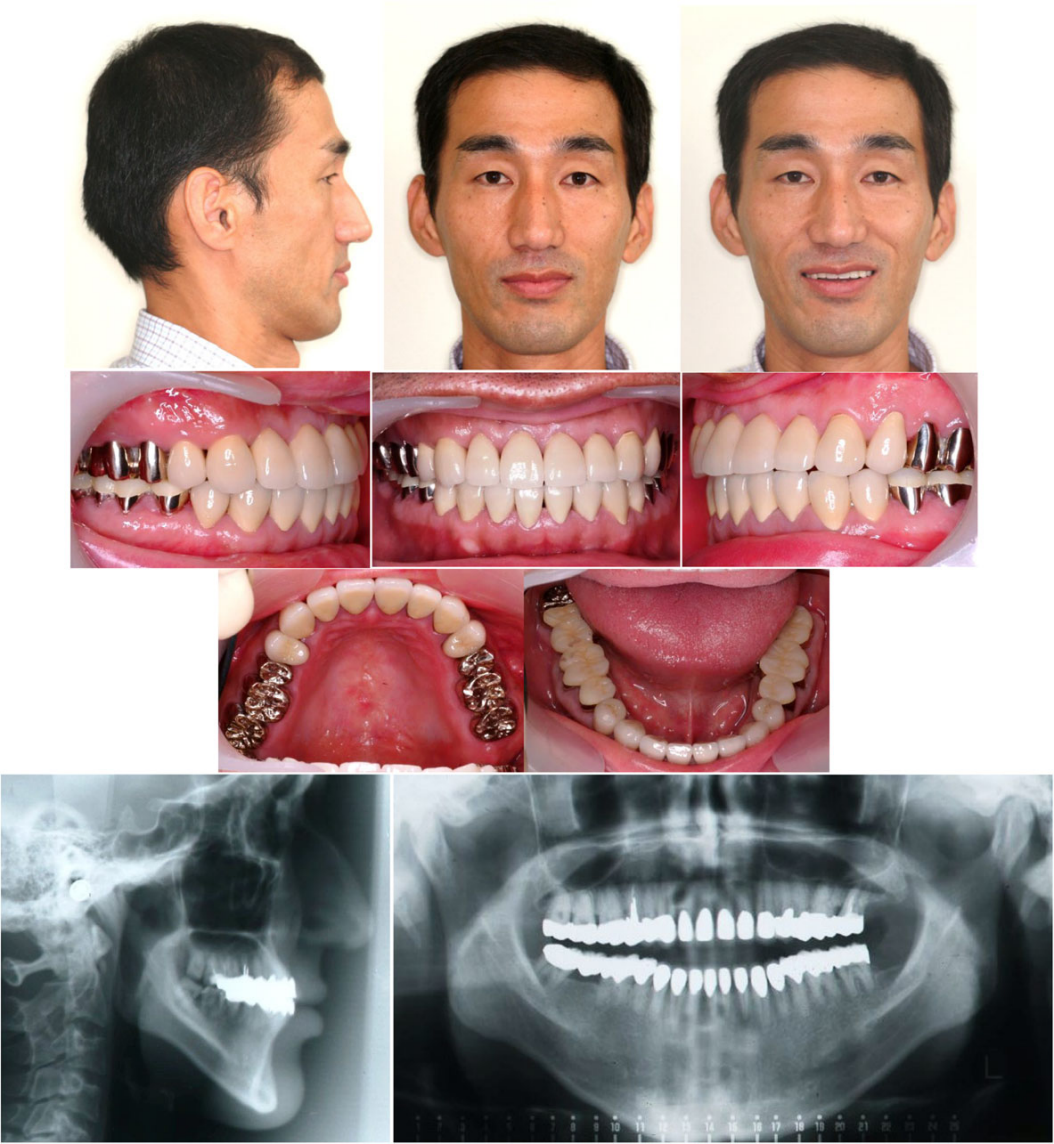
Posttreatment facial and intraoral photographs, lateral cephalogram and panoramic radiograph
